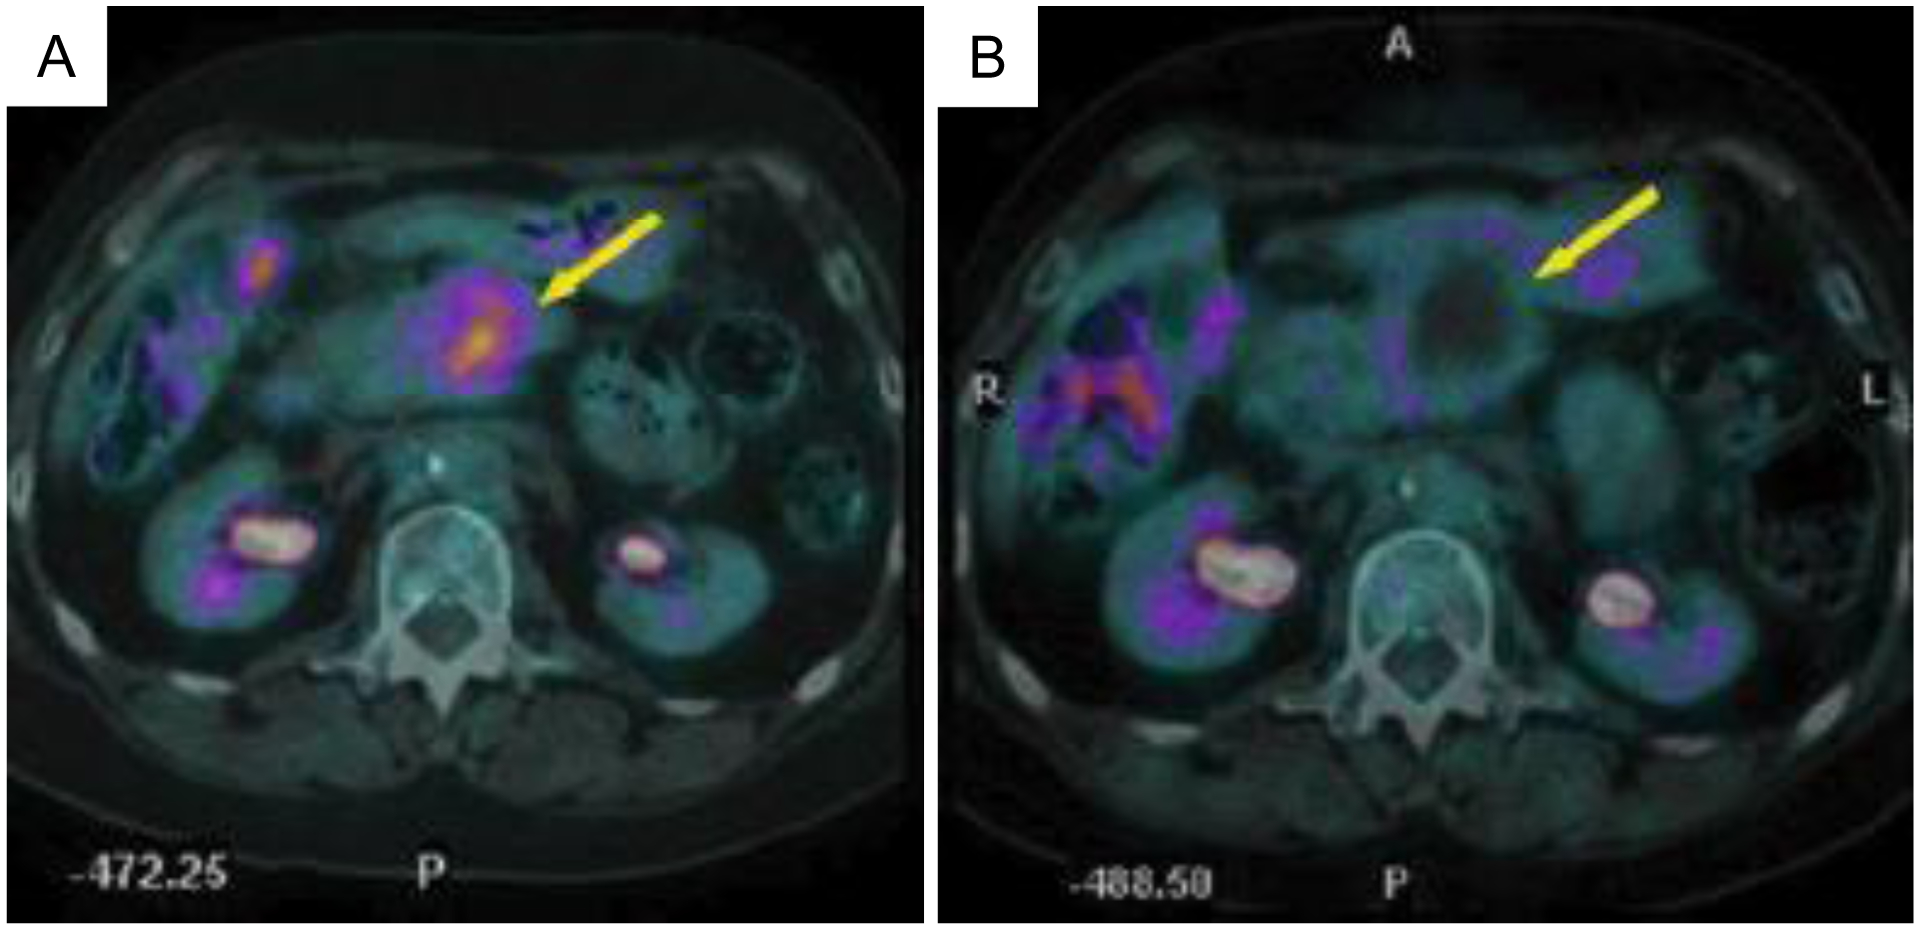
^18^F-FDG PET pre and postthermal HIFU ablation in the pancreas. Transverse view of positron emission tomography computed tomography (PET-CT) images from a 60-year-old patient with pancreatic cancer before and after thermal ablation. (a) Pretreatment PET-CT image showing tumor fluorodeoxyglucose uptake (bold arrow). (b) One month after HIFU, the PET-CT image shows lack of metabolic activity (bold arrow). Figure adapted from [15].
